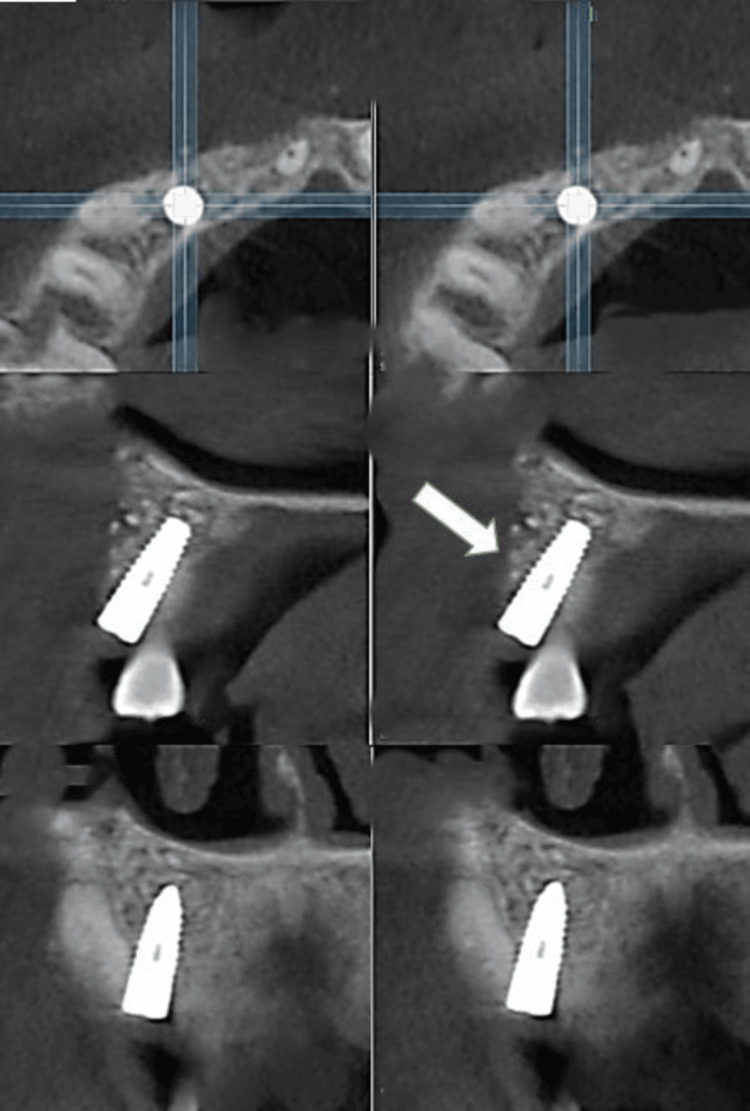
Sagittal view of the post-operative CBCT scan showing the osseointegrated implant CBCT: Cone beam computed tomography
